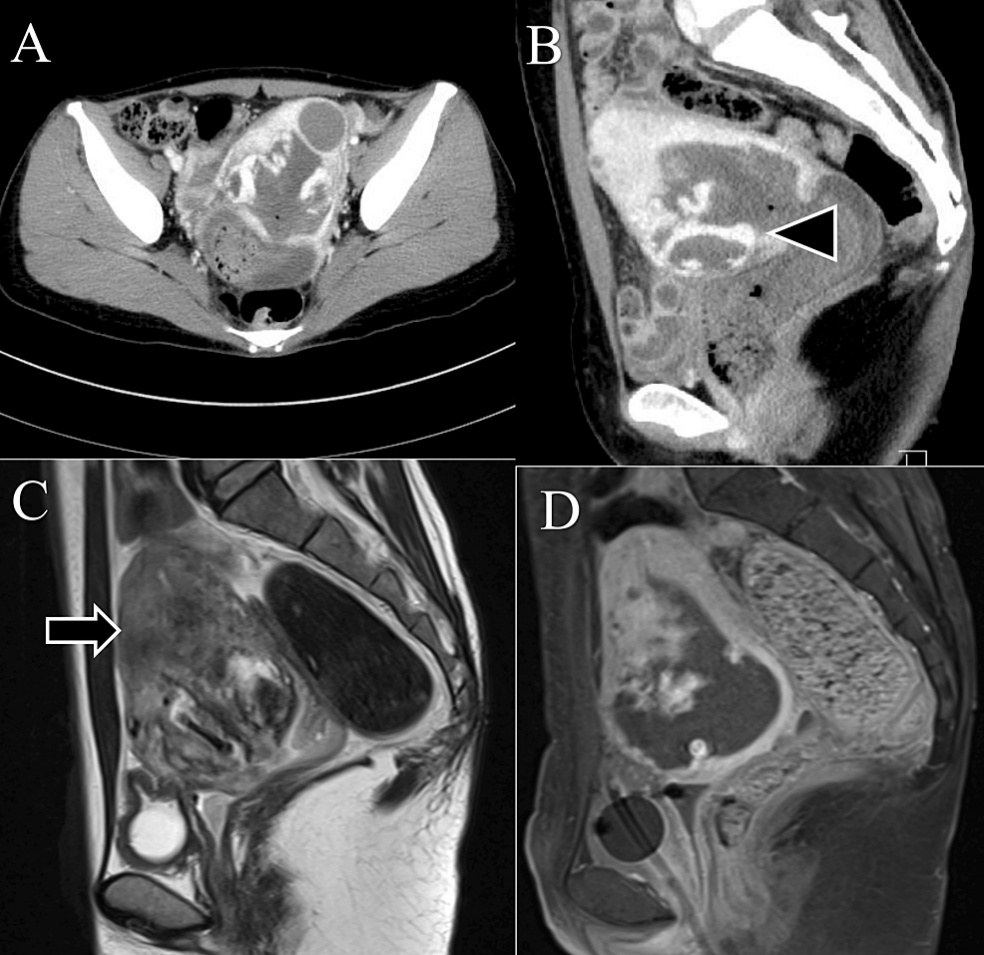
Contrast-enhanced CT and MRI A) and B) show the contrast-enhanced computed tomography images performed on postpartum day 44. A hypervascular lesion was observed within the myometrium(head arrow), suggesting the possibility of a pseudoaneurysm or hypervascular RPOC. C) and D) show the magnetic resonance images on postpartum day 44. Large remnants were visualized in the uterine cavity, suggesting the possibility of RPOC. The junctional zone had disappeared(arrow), and vascular infiltration into the myometrium was confirmed (C and D).
